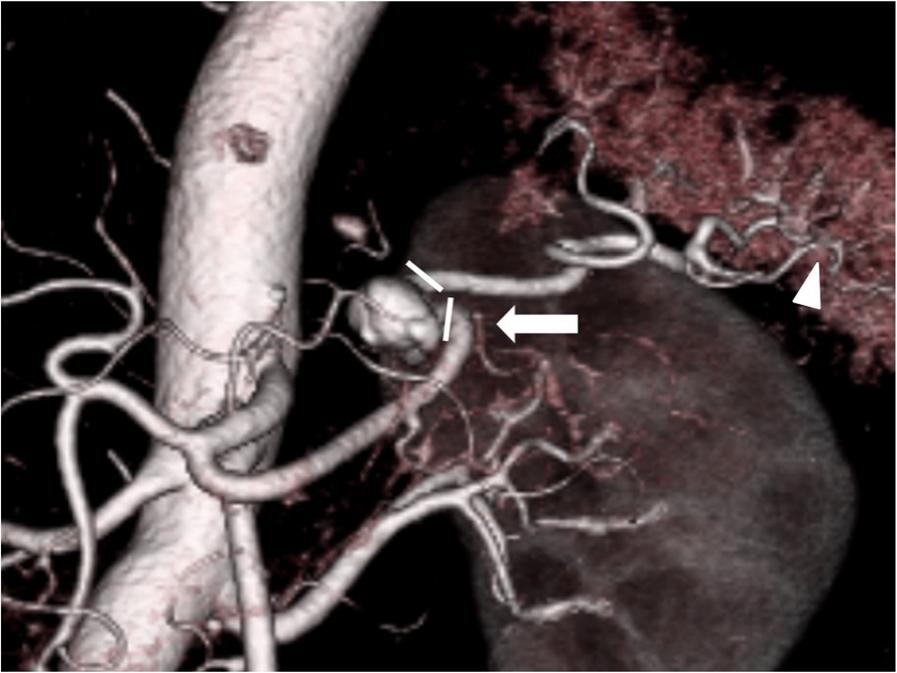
Preoperative enhanced computed tomography. Enhanced computed tomography reveals a 10-mm aneurysm in the middle third of the splenic artery. *White triangle: omental branches of the left gastroepiploic artery via the omental artery; white arrow: the great pancreatic artery via the pancreas; white lines: the range where the splenic artery was resected
